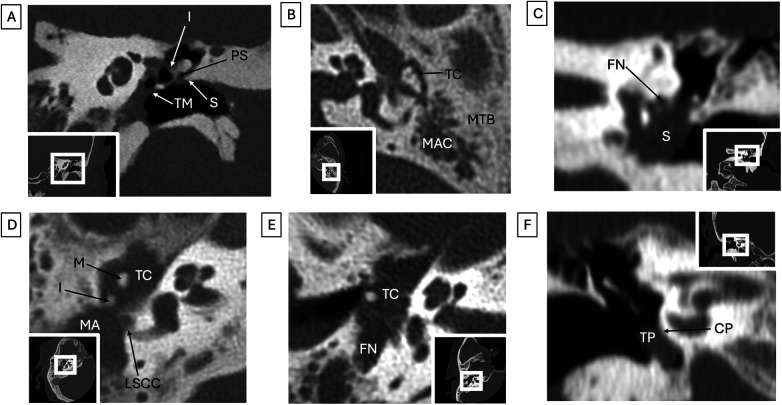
Middle ear pathology (all images represent different patients). (A) Cholesteatoma. Photon-counting coronal CT shows soft tissue opacification within the epitympanum, including involving Prussak's space (PS), and with erosion of the medial aspect of the incus (I) and retraction of the tympanic membrane (TM). No erosion of the scutum (S). (B) Chronic otitis media. Axial CT shows complete opacification of the left tympanic cavity (TC) and mastoid air cells (MAC) with marked sclerosis of the mastoid part of the temporal bone (MTB). (C) Facial nerve schwannoma. Coronal CT shows an intratympanic soft tissue mass (S) which contacts the tympanic facial nerve canal (FN). (D) Metastasis. Axial CT shows a soft tissue mass filling the tympanic cavity (TC) and mastoid antrum (MA) with erosion and dissociation of the malleus (M) and incus (I) as well as dehiscence of the lateral semicircular canal (LSCC) in a patient with metastatic non-small cell lung cancer. (E) Chronic idiopathic polyneuropathy. Axial CT shows marked expansion of the mastoid facial nerve canal (FN) with remodeling, expansion, and soft tissue opacification of the tympanic cavity (TC) due to enlargement of the facial nerve and chorda tympani. (F) Tympanic paraganglioma. Coronal CT shows an ovoid soft tissue density (TP) within the tympanic cavity abutting the cochlear promontory (CP) near the expected location of the tympanic branch of cranial nerve IX.
